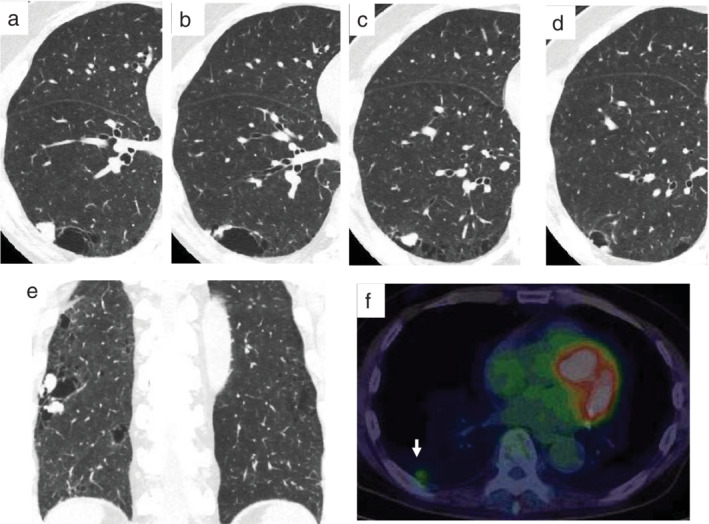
Diagnostic imaging. (a–d) Serial axial section images in a lung window setting on computed tomography showed a thin‐walled cystic airspace with exophytic and endophytic solid nodules along the cyst wall in the right lower lobe. (e) A coronal section image showed several multisided nodules abutting the wall of a multilocular cystic airspace in the emphysematous lung. (f) 18F‐FDG–positron emission tomography showed marginal FDG uptake, with a maximum standardized uptake value of 2.6 in the lung nodule (arrow). FDG, fluorodeoxyglucose.
